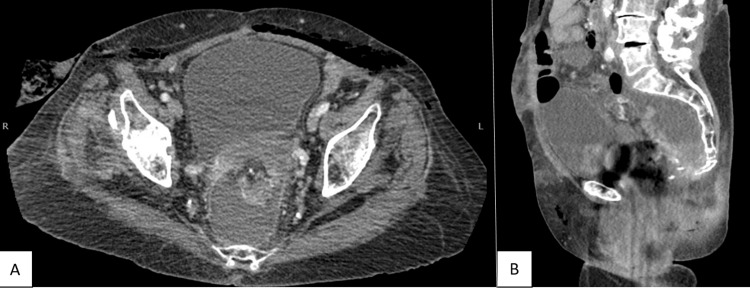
CT scan of the abdomen and pelvis two weeks after surgery. There was a fluid collection, representing an abscess cavity, posterior to the anastomosis. (A) Axial view showing the width of the collection. (B) Sagittal view showing the proximal extent of the collection.
